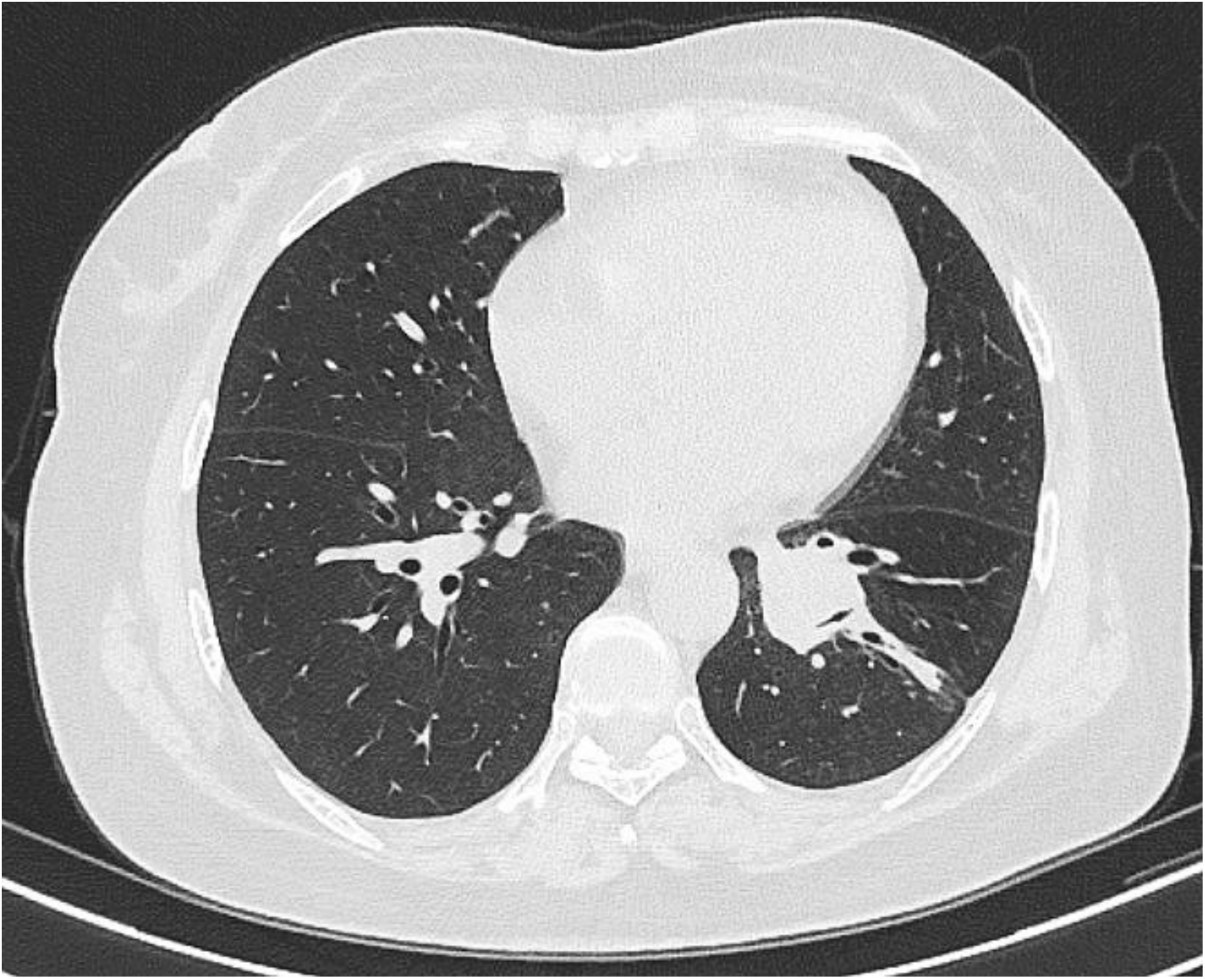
16-slice computerized tomography images of the reexamination of chest CT showed the lesion significant reduction.
